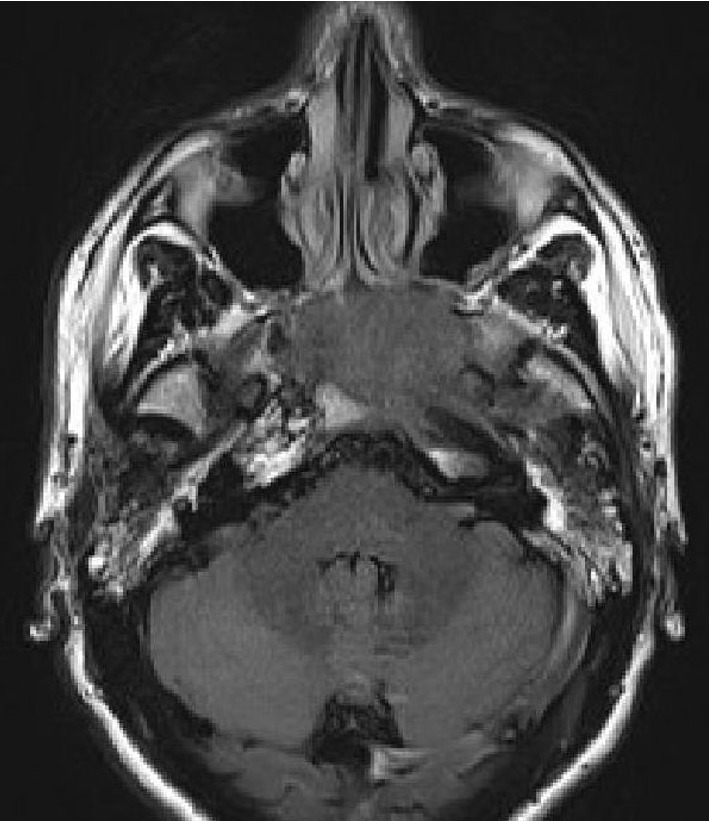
Magnetic resonance imaging of the head and neck revealed a nasopharyngeal mass with extension into the left pterygopalatine fossa. Bony involvement of the greater wings of the sphenoid and clivus was described.
